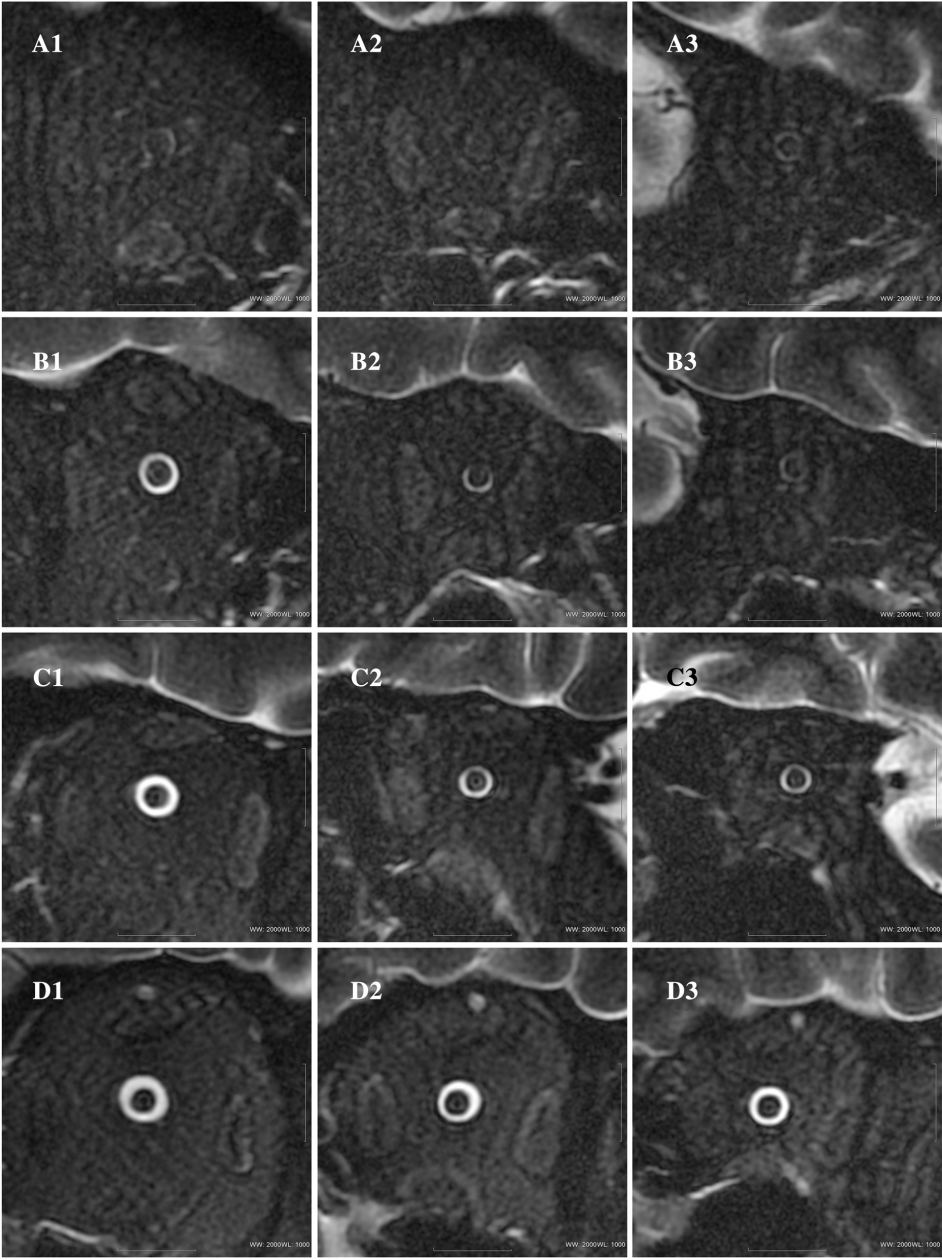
Oblique magnetic resonance image of the optic nerve/sheath complex (coronal T_2_-weighted fast-recovery fast spin-echo sequence (T2WI-FRFSE) with fat suppression; digital field of view = 4, window width = 2,000, window level = 1,000), taken at 3 mm (Figure A1, B1, C1, and D1), at 9 mm (Figure A2, B2, C2, and D2), and at 15 mm (Figure A3, B3, C3, and D3) behind the globe. Figure A1 to 3: A 70-year-old woman with spontaneous intracranial hypotension; lumbar ICP value was 3.7 mm Hg. Figure B1 to 3: A 39-year-old man with head injury; lumbar cerebrospinal fluid pressure was 8.5 mm Hg. Figure C1 to 3: A 33-year-old man with peripheral neuropathy; lumbar cerebrospinal fluid pressure was 16.2 mm Hg. Figure D1 to 3: A 40-year-old man with idiopathic intracranial hypertension; lumbar cerebrospinal fluid pressure was 24.3 mm Hg.
